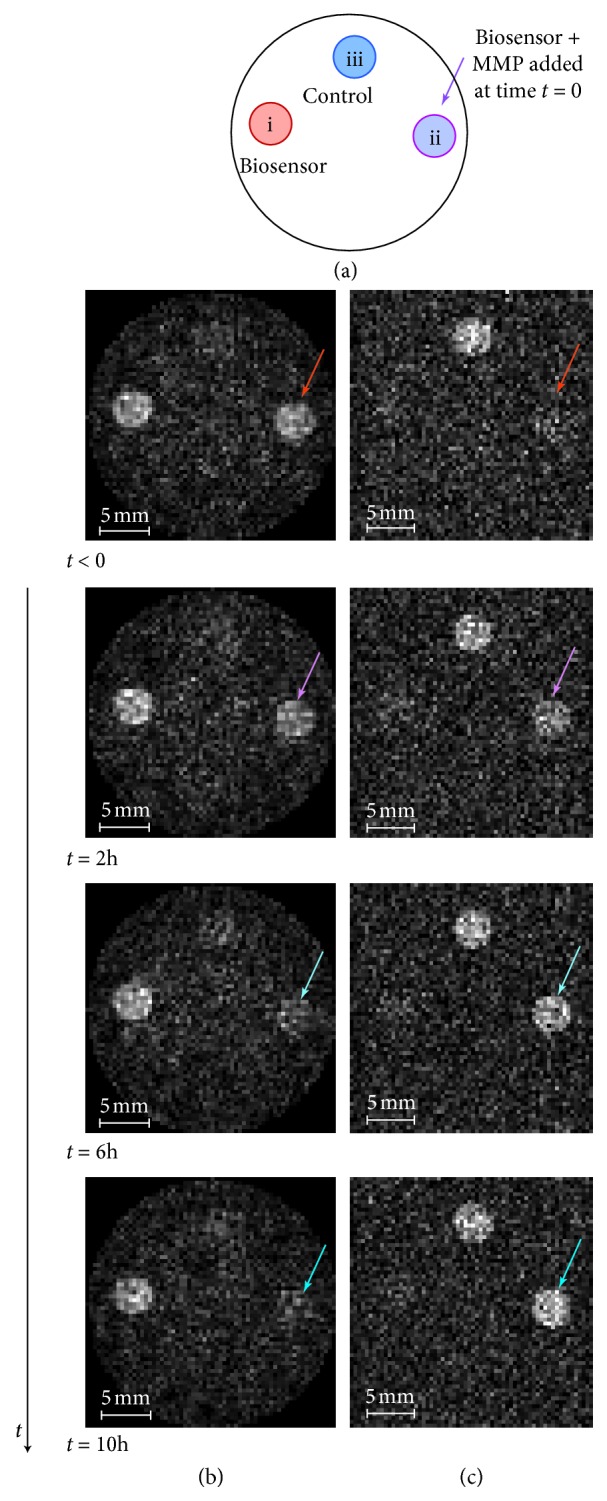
Transverse ^19^F·MRI at 9.4 Tesla and 293 K of three separate 5 mm NMR tubes (located within a 30 mm microimaging probe head), each containing 600 *μ*L of 1.2 mM solution of the respective ^19^F samples in 9 : 1 v/v H_2_O/acetonitrile solution, were used to demonstrate the effect of negative *T*
